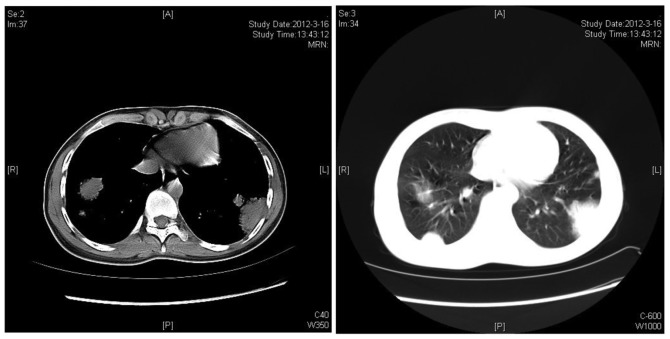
Bilateral pulmonary consolidation on computed tomography.
